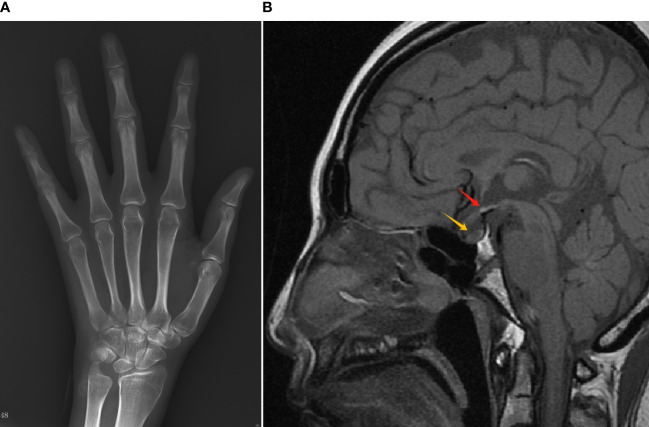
Radiology results of the patient. (A) X-ray of the left hand/wrist showed epiphyseal closure. (B) MRI of the sella turcica showed a small anterior pituitary remnant (yellow arrow), invisible pituitary stalk, and an ectopic neurohypophysis (red arrow).
